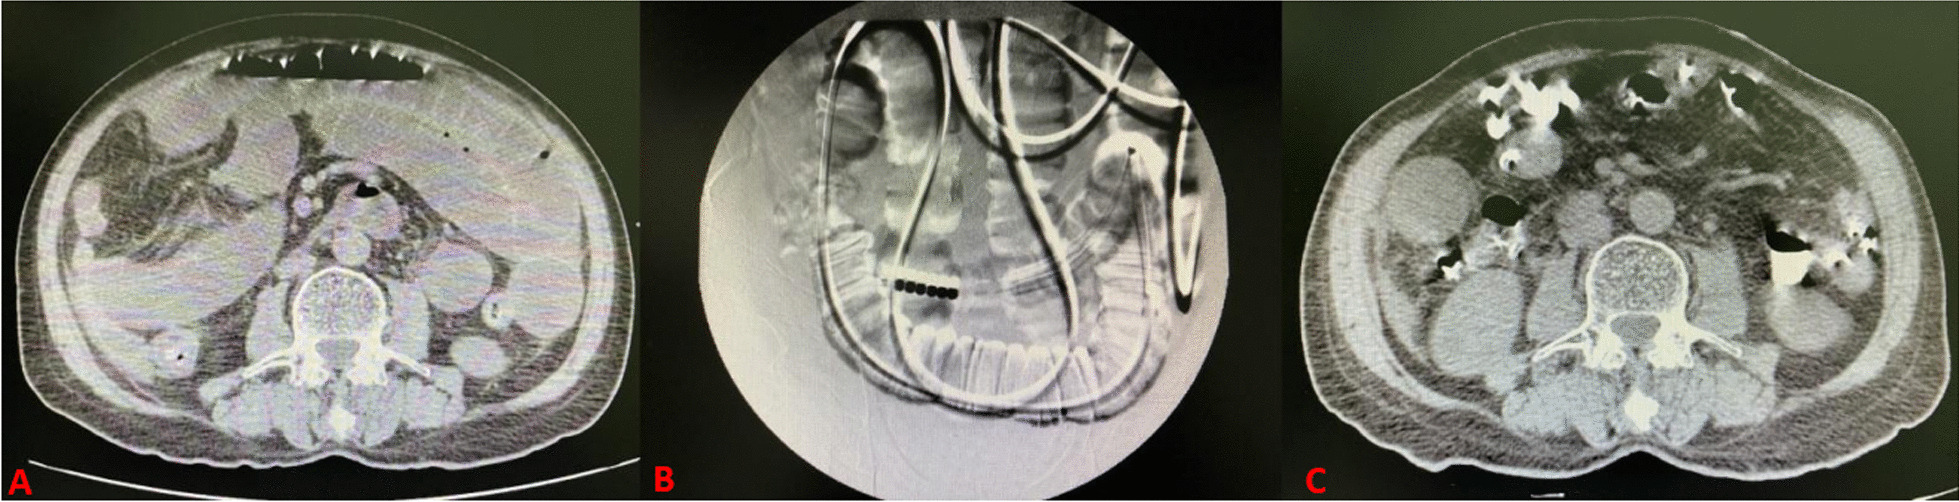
CT image of a patient with adhesive ileus with successful decompression after NIT insertion. A Before NIT insertion; B X—ray of completion of NIT insertion; C Three days after NIT insertion
